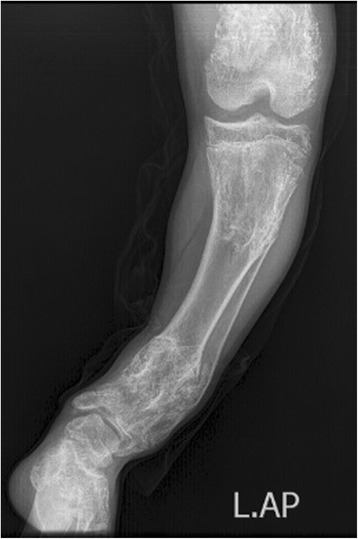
Anterior posterior x-ray of left leg: lytic lesions in the proximal and distal metaphyses of left tibia and fibula
